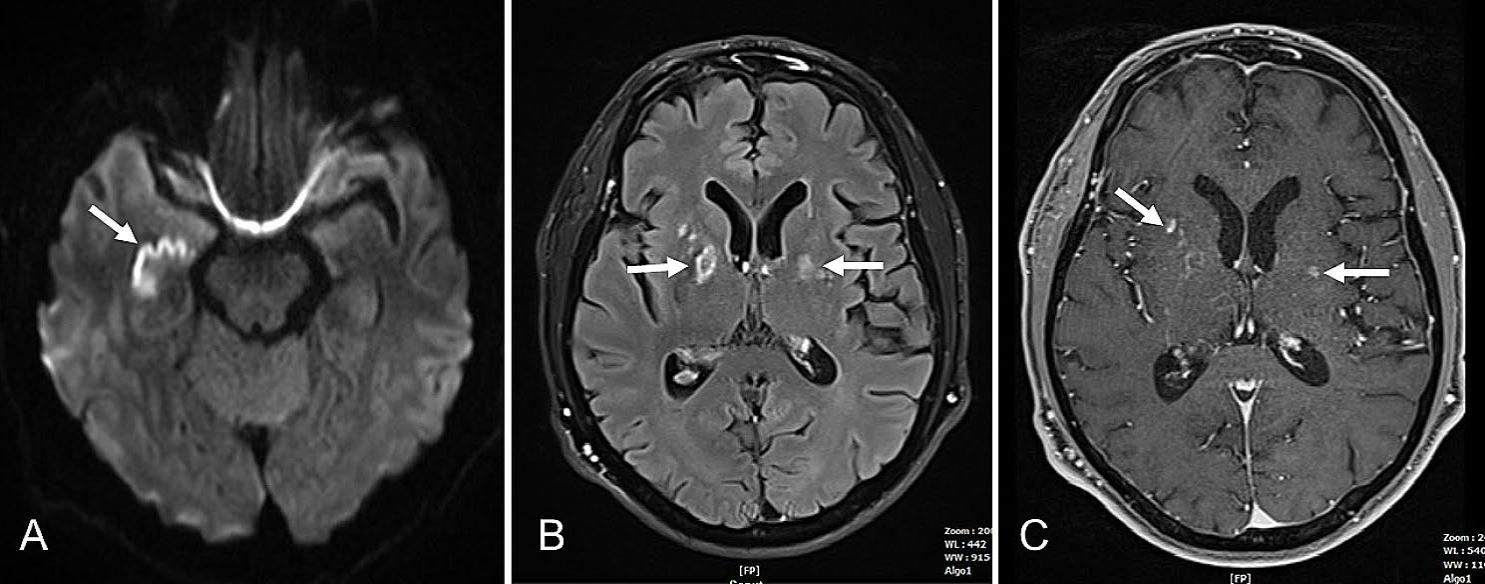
A-C: MRI scan on day 6. DWI (A), FLAIR (B) and contrast enhanced T1w images (C), disclosing restricted hippocampal diffusion (A) and basal ganglia lesions (B) with contrast enhancement (C)
